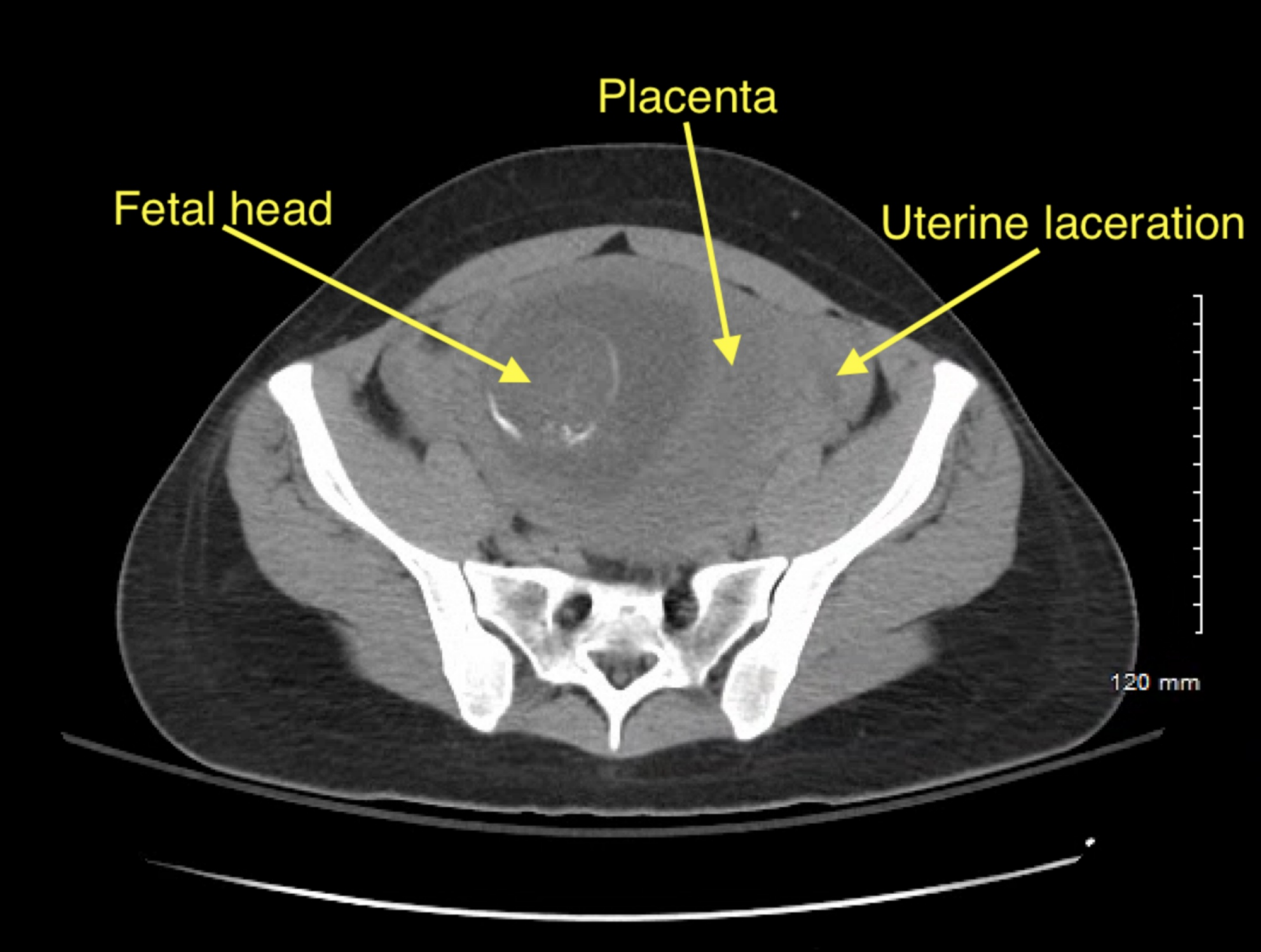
Transverse CT of pelvis with fetus within uterine cavity.
